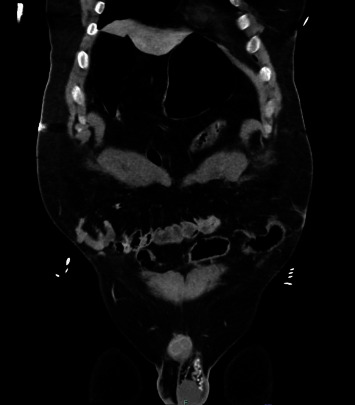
Abdominal CT. Bilateral transection of rectus abdominis musculature (arrows) with resultant bowel herniation.
